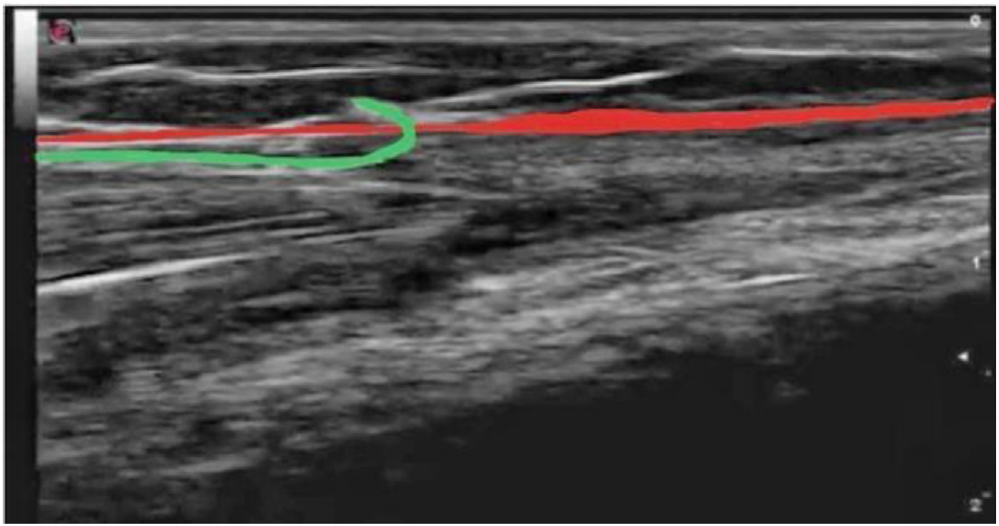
Ultrasound picture of hook dividing fascia (green). The hyperechoic fascia is indicated in red.
